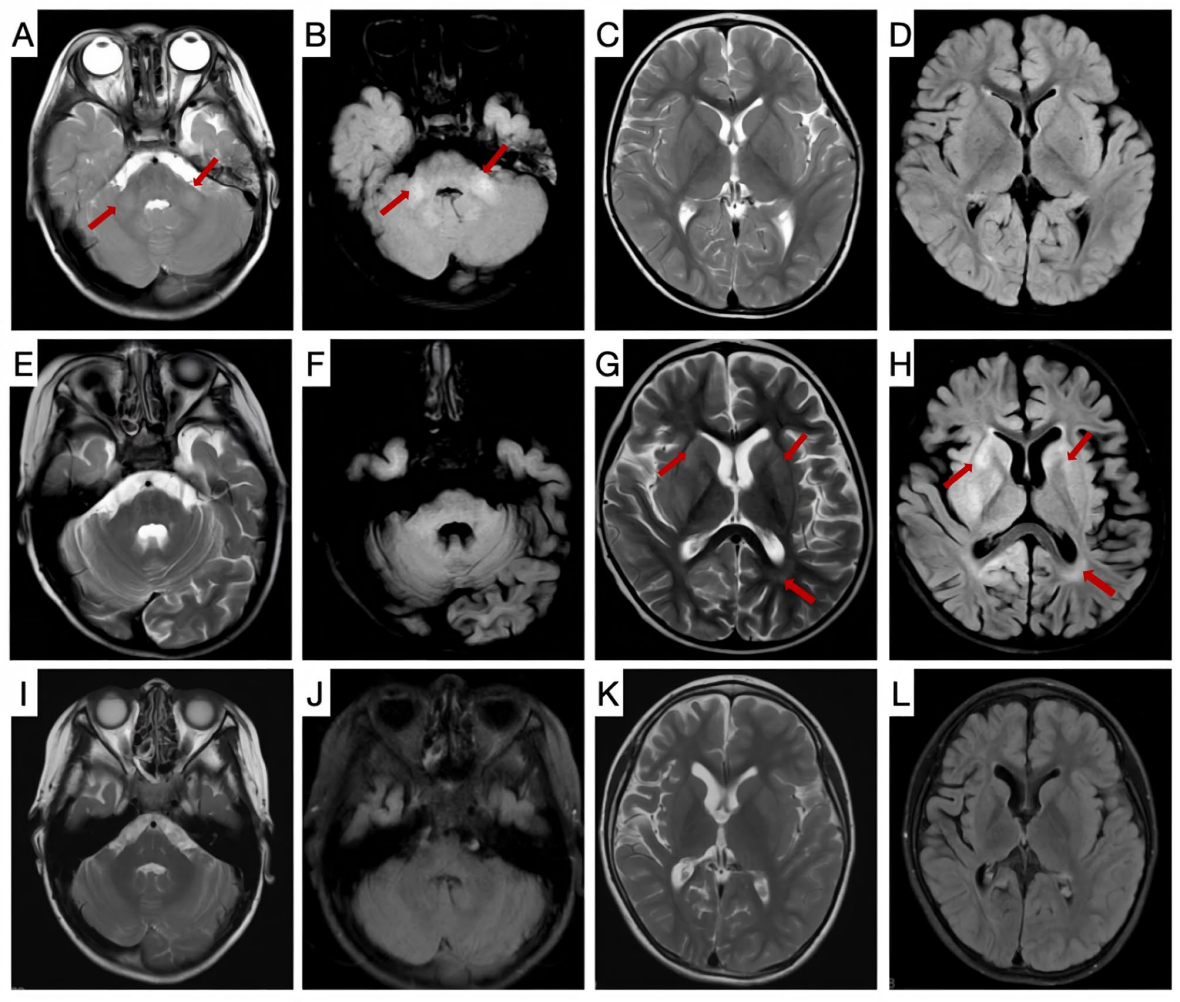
Cranial MRI images of patients with NAXE deficiency. Patient 1 was a 7-year-old girl with a homozygous variant in the NAXE gene (c.733 A > C), who first presented with gait instability at 2 years and 7 months of age. Cranial MRI revealed abnormal signals in the bilateral cerebellar dentate nuclei on T2 and T2 FLAIR sequences (A-D). After treatment with hormones combined with intravenous immunoglobulin, her symptoms resolved to baseline. Two months later, the patient developed recurrent gait instability and altered consciousness following fever. Repeat MRI (T2 and T2 FLAIR sequences) showed new abnormal signals in the bilateral basal ganglia and periventricular white matter of the posterior horn of the lateral ventricles (E-H). Nicotinamide therapy was initiated at 5 months of disease onset, and symptoms gradually improved. A follow-up cranial MRI 3 years and 4 months after treatment showed significant improvement in abnormal signals in the cerebellar dentate nuclei, basal ganglia, and periventricular white matter of the posterior horn of the lateral ventricles, but left brain atrophy predominantly in the right cerebral hemisphere (I-L)
